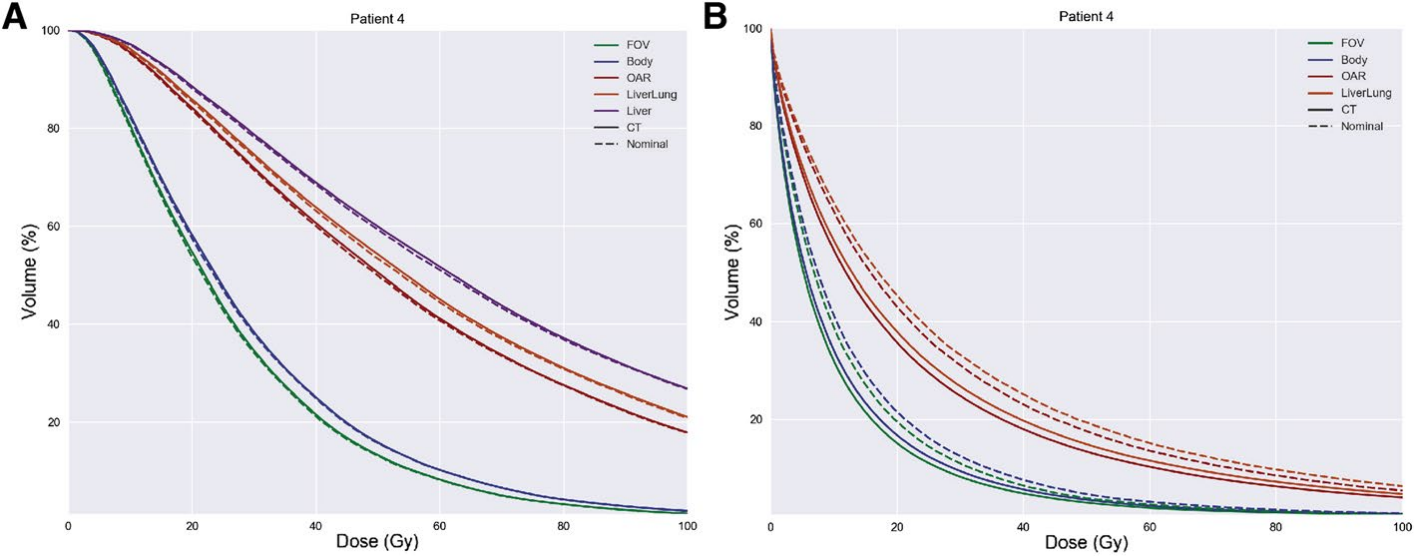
Liver (A) and lung (B) cumulative dose volume histograms (DVHs) are shown for one patient. Each calibration includes the DVH calculated using the CT and nominal local deposition methods
